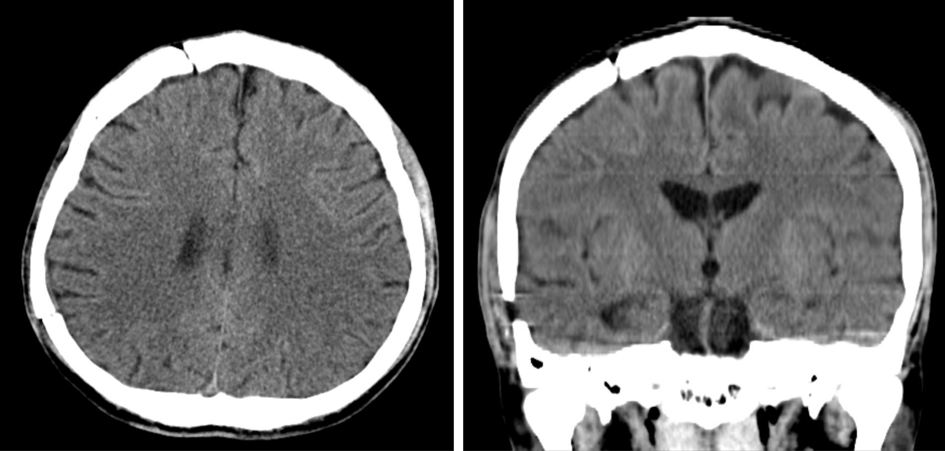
CT of the head, 40 days after cranioplasty. Left, axial view; right: coronal view. CT, computed tomography.
